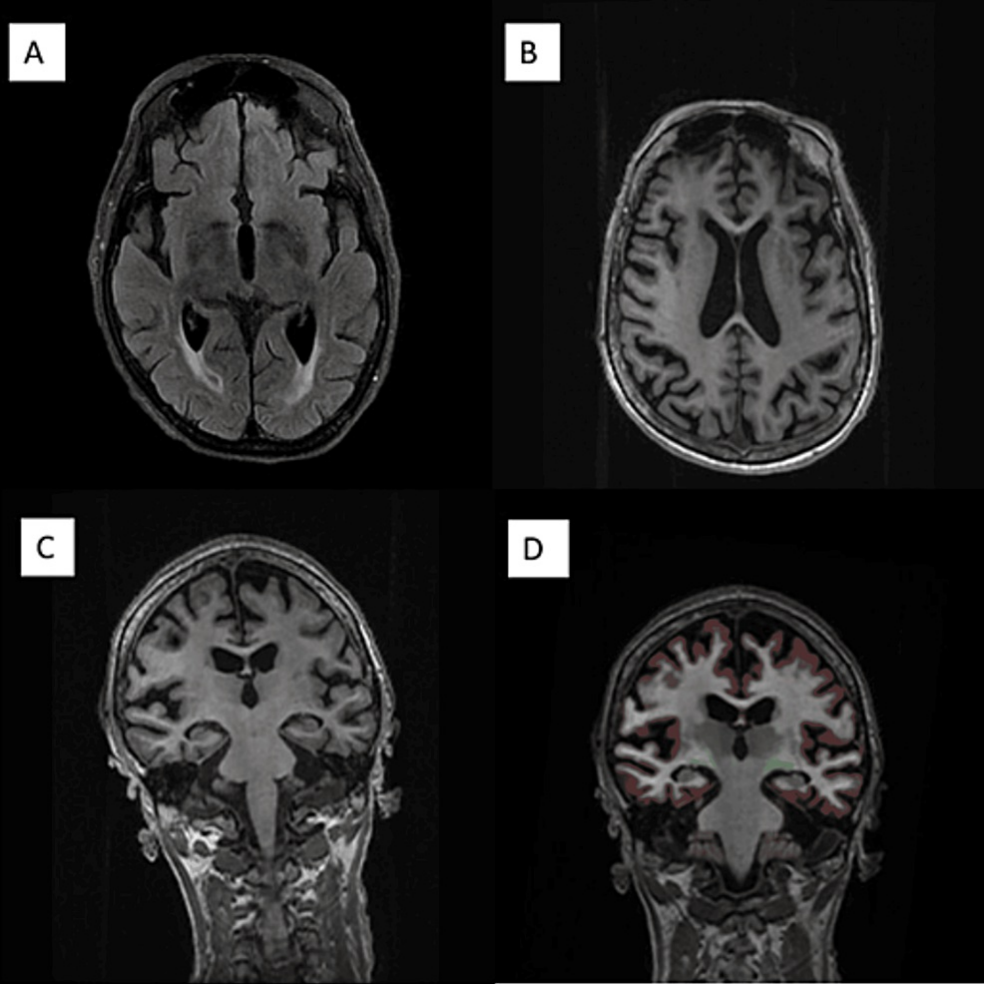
MRI brain with and without contrast at nine-month post-symptom onset (A) Axial T2 FLAIR images demonstrating nonspecific peri ventricular ischemic changes. (B) Axial T1 post-contrast images showing no evidence of enhancement. (C and D) Coronal T1 and NeuroQuant MRI demonstrating statistically significant hippocampal atrophy
